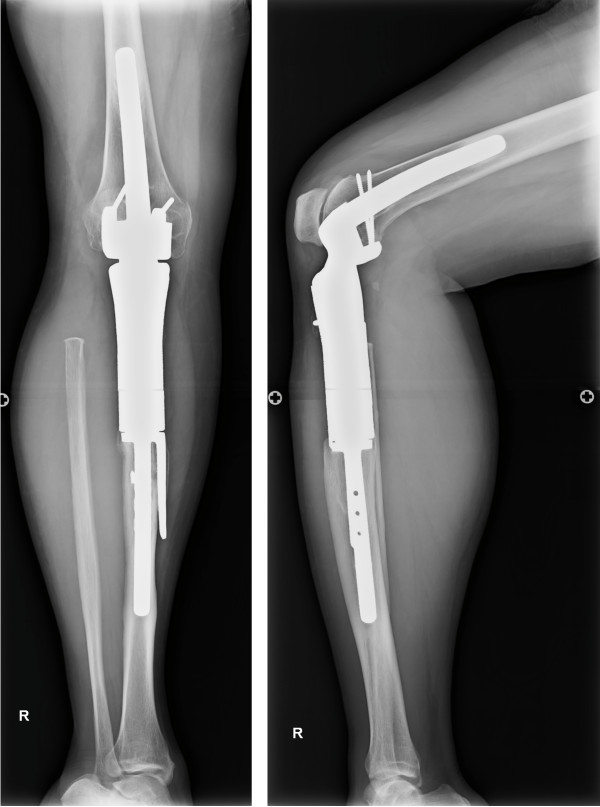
Postoperative radiographs of tibial adamantinoma. Anteroposterior and lateral radiographs show the uncemented megaprothesis (Howmedica Modular Replacement System) used to treat the patient after prior resection of an adamantinoma of the right tibia. No evidence of recurrence is present.
